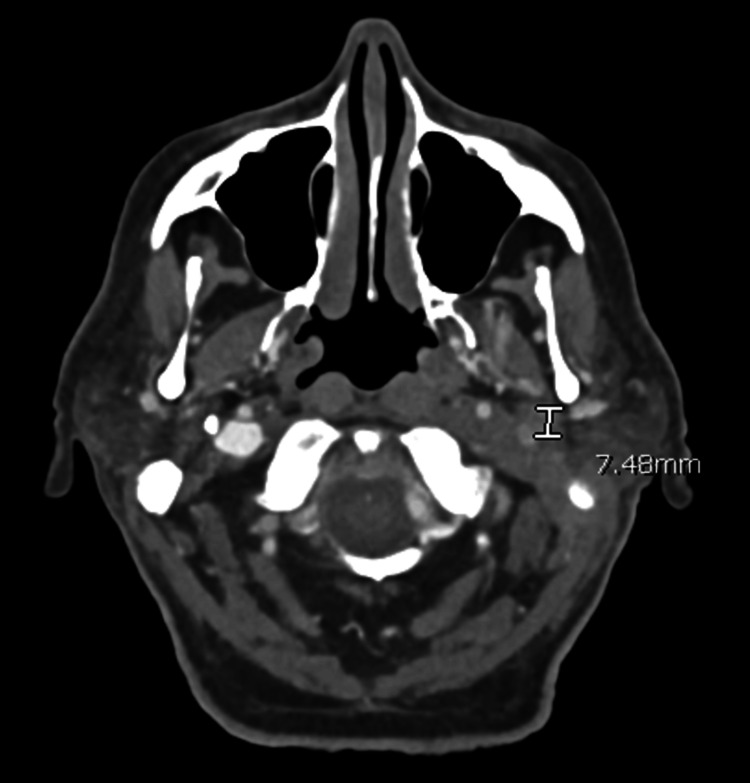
Computed tomography (CT) scan of the neck with a transverse view showing short-segment narrowing of the superior left internal cervical carotid artery.
